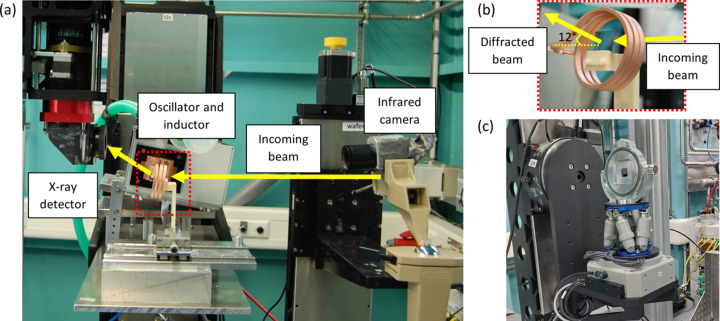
XWBT setups used for the measurements at the KIT Light Source. (*a*) *In situ* sample environment installed on a translation stage. The X-ray detector and the IR camera observe the sample simultaneously. (*b*) The sample positioned in the center of the induction coil. (*c*) *Ex situ* setup enabling flexible sample positioning and orientation.
